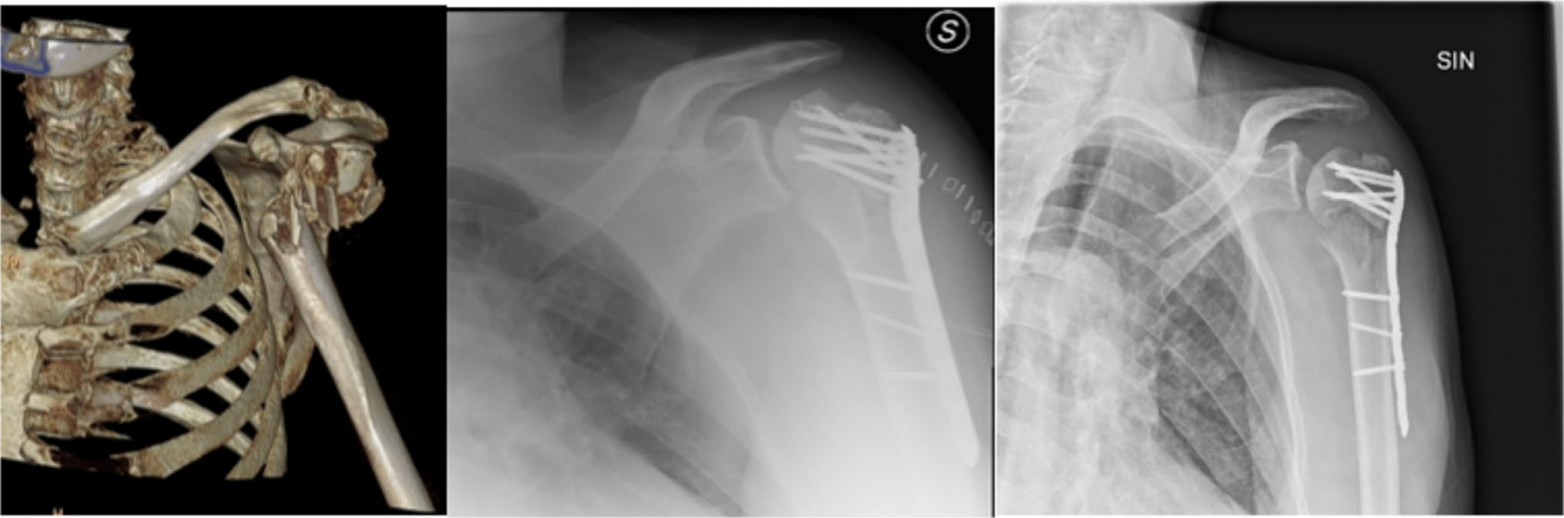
3D-TC and X-rays of a comminuted proximal humerus fracture in a 75-year-old man, pedestrian hit by a car. Inadequate restoration of both the medial hinge and the cervico-dyaphyseal angle, finally leading to AVN after 8 months post-op
